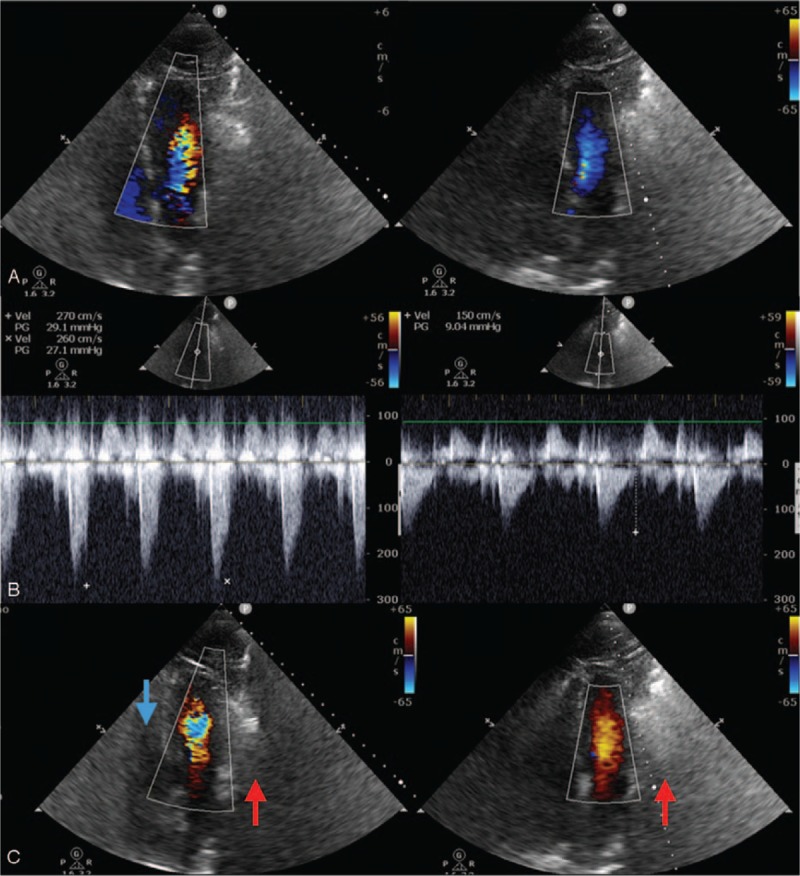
Echocardiographic imaging studies before (left panel) and after treatment (right panel). In a small hyperdynamic LV cavity, an aliasing of color flow doppler images was found across LVOT (panel A, left panel) with a maximal pressure gradient of 29 mmHg estimated by a continuous-wave doppler (panel B, left panel) in systole, and a paradoxical dynamic obstruction with a jet flow from the apex LV chamber to the basal was recognized in diastole (panel C, left panel) (video in Supplement 3). At post-treatment, the LV cavity enlarged with a laminar LVOT blood flow in systole (Panel A, right panel) and a laminar LV cavity blood flow from base to apex in diastole (Panel C, right panel) (video in Supplement 4). Meanwhile, the LVOT pressure gradient decreased to 9.0 mmHg (Panel B, right panel) in systole. LV = left ventricular, LVOT = left ventricular outflow tract.
